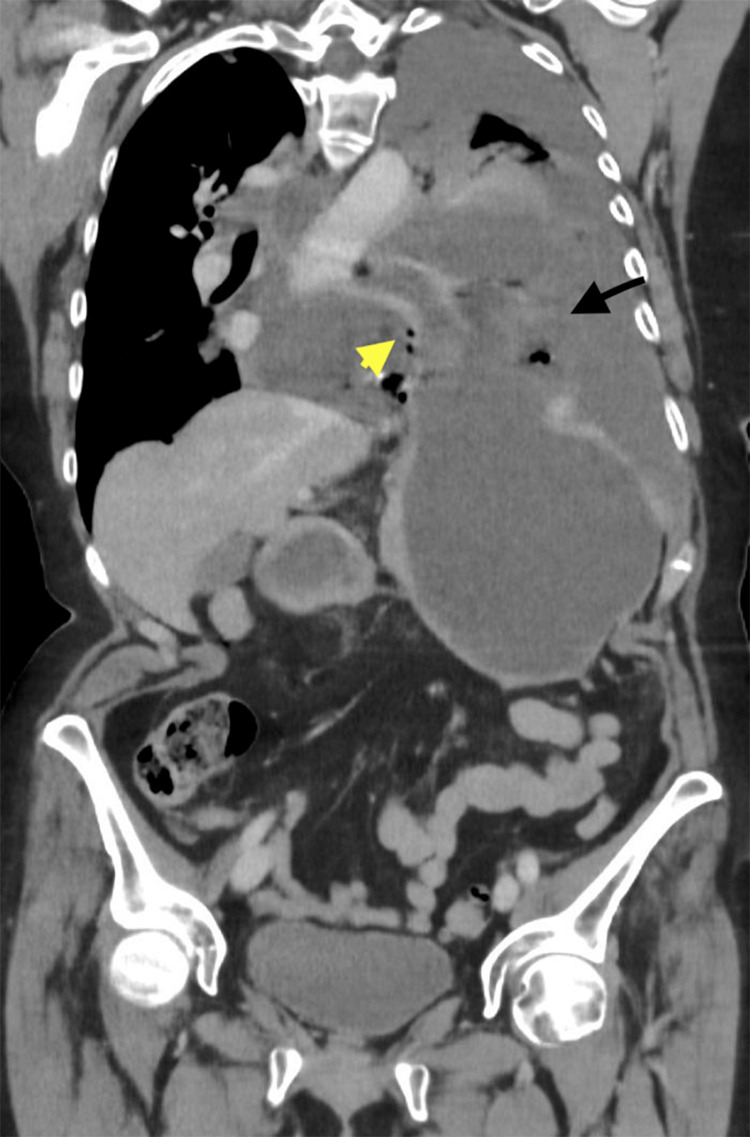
Coronal contrast-enhanced CT image of the thorax A large intrathoracic paraesophageal hernia is seen. A focal absence of gastric wall enhancement (black arrow) is observed, consistent with severe vascular compromise and transmural ischemia. Additionally, free intrathoracic air (yellow arrow) is present, indicating perforation. These findings are highly suggestive of strangulation of the herniated stomach with subsequent necrosis and perforation.
